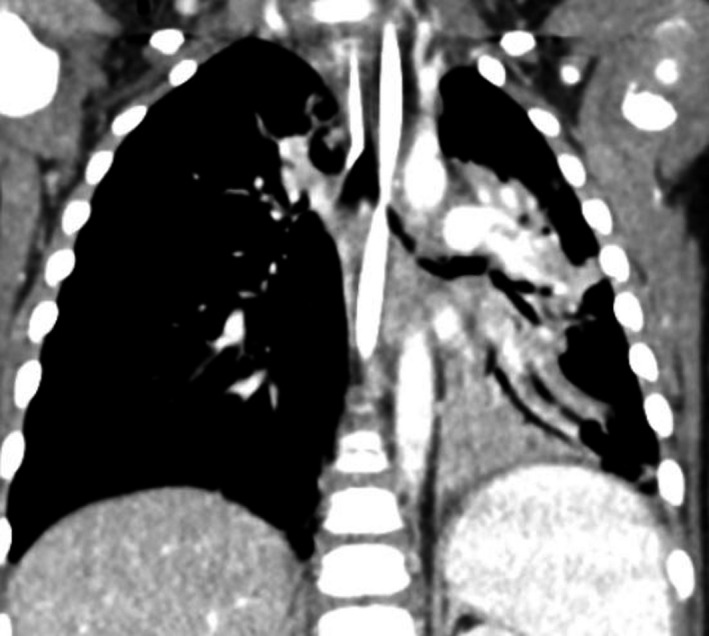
CT of chest with contrast. Overall radiographic interpretation identified narrowing of the left main stem bronchus containing a transverse dimension irregular filling defect. The left lung shows marked volume loss. There is also collapse of the left upper lobe segment. No vascular ring or sling is identified. In this section, you can also visualize significant left lower lobe involvement
